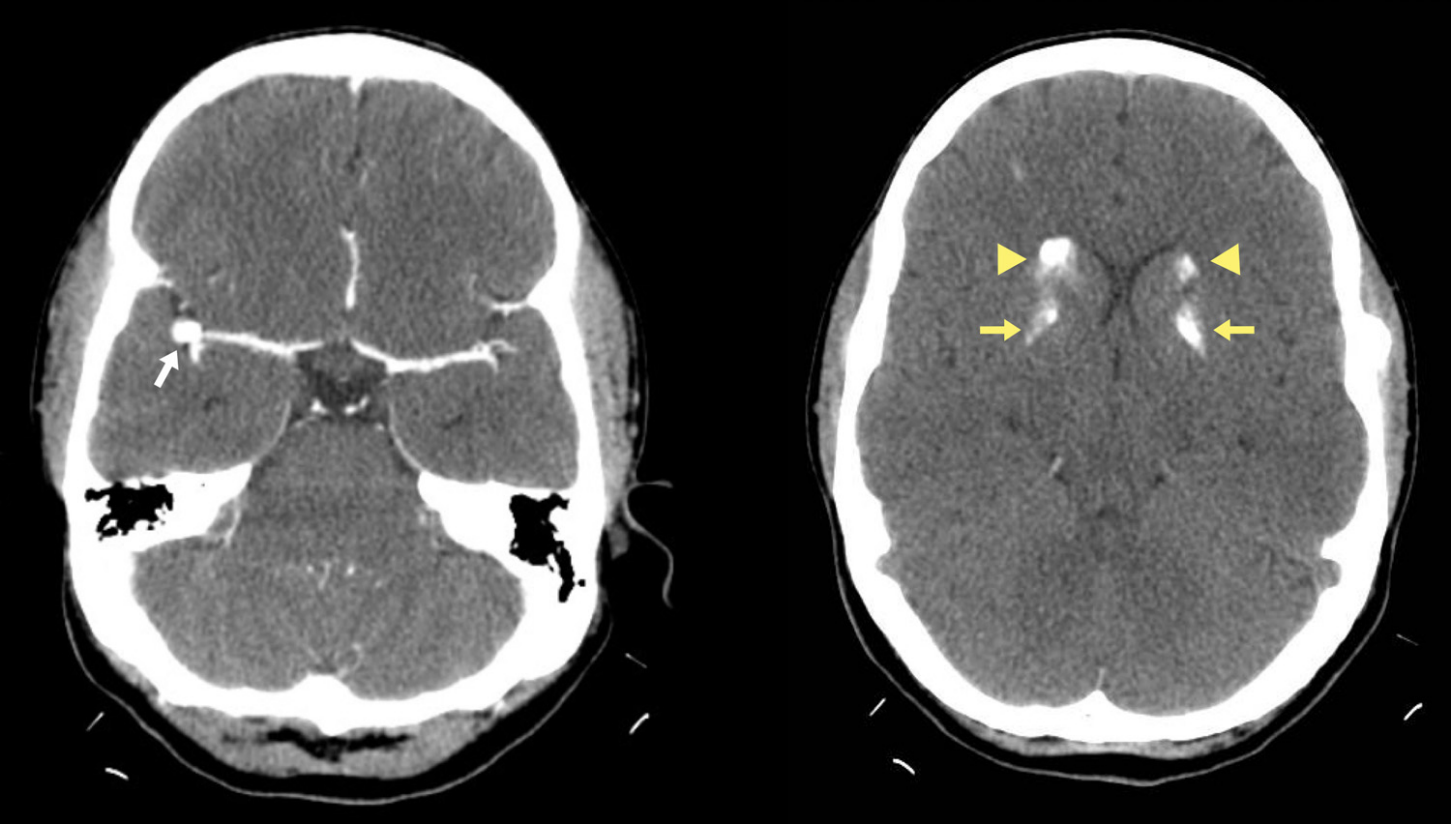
Contrast-enhanced head CT: showing an aneurism of the right middle cerebral artery at the angle of M2-M3 (Fig. 1A). Incidentally, bilateral and symmetric calcifications of the caudate and lenticular nuclei compatible with Fahr disease were noted.
